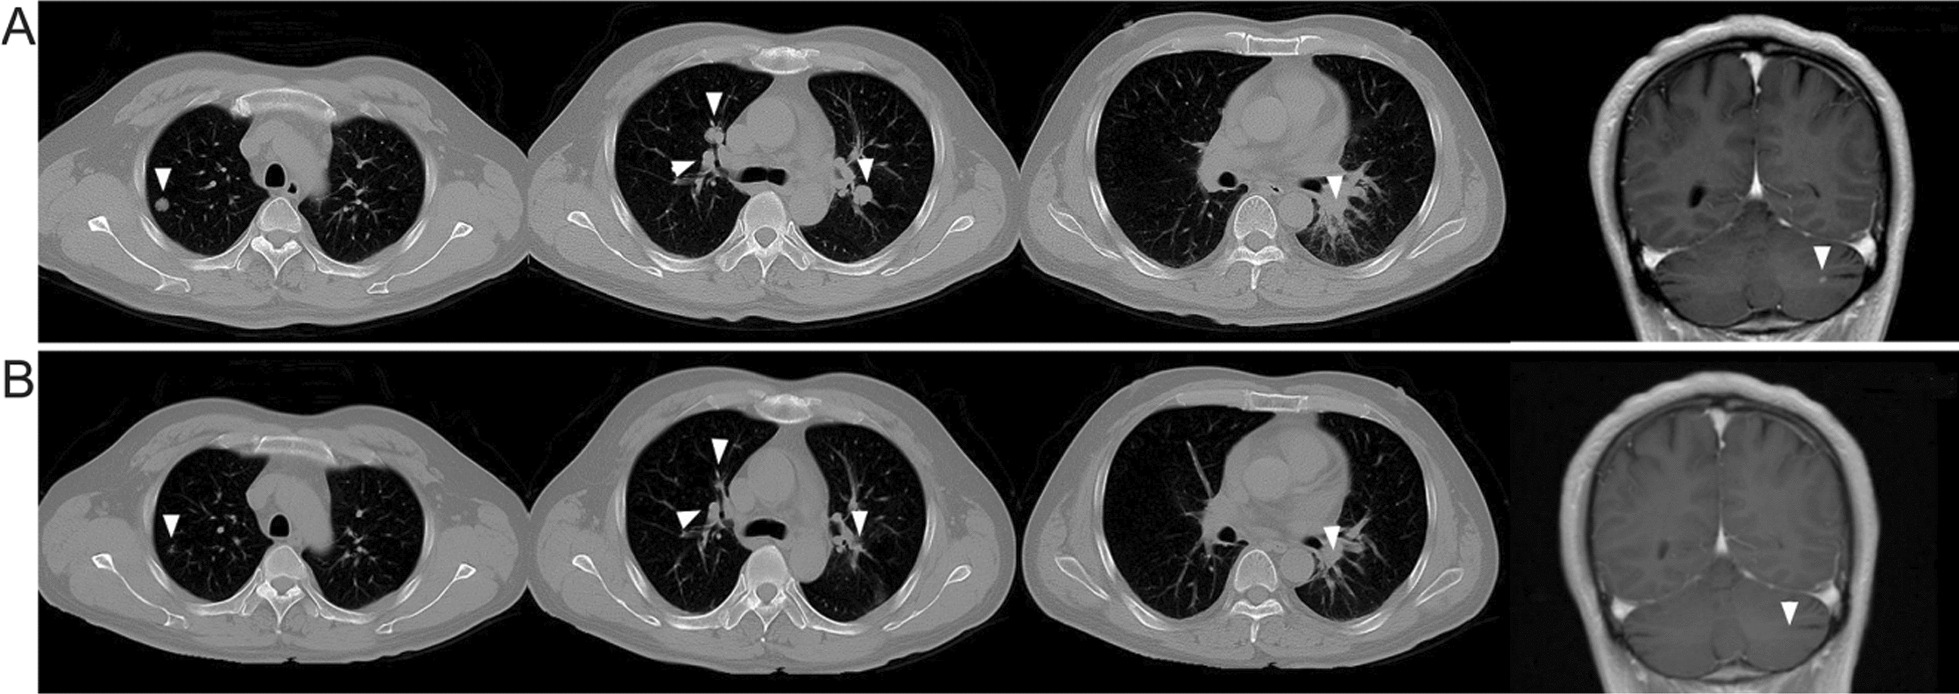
The chest and brain CT scan of before and after Osimertinib treatment. A Multiple pulmonary nodules and a cerebellar lesion had appeared in the CT scan, which indicated disease relapsed at 20 months after the initial diagnosis (white arrows). By this time the patient also received additional chemotherapy of Pemetrexed and cisplatin. EGFR-T790M is detectable in the peripheral blood. B Osimertinib treatment resulted in a partial response of the pulmonary lesions and complete response of the cerebellar lesion.
